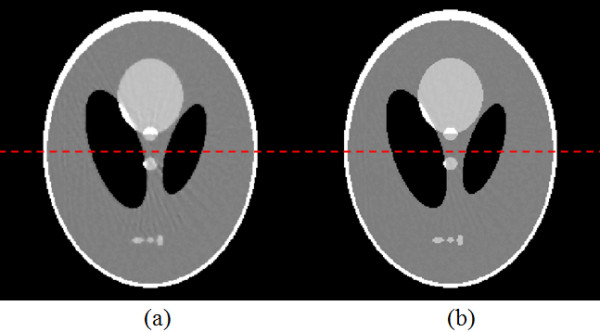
A comparison study on the reconstructed images by ASD-POCS and API-TV algorithms respectively, each of which took 60 additive noisy cone-beam projections (about 10% white noise) and 50 iterations: (a) the reconstructed image by the ASD-POCS algorithm, and (b) the reconstructed image by the proposed API-TV algorithm. The grey scale display range is [0.8, 1.2].
